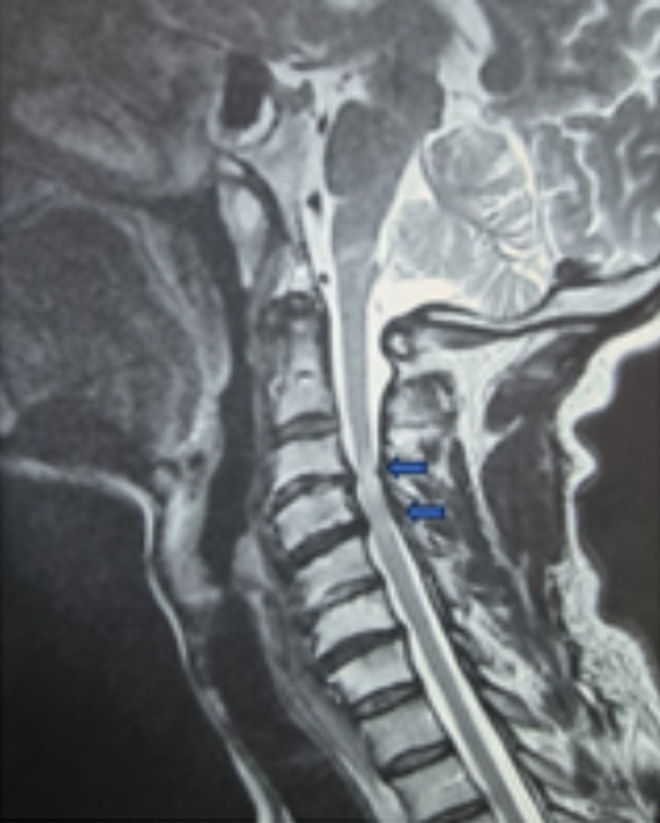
magnetic resonance imaging scan displays two cervical disc herniations at C3-C4 and C4-C5, associated with disco-osteophytic spurs; arrows indicate the location of the disc herniations and thinning of the peri-medullary space
